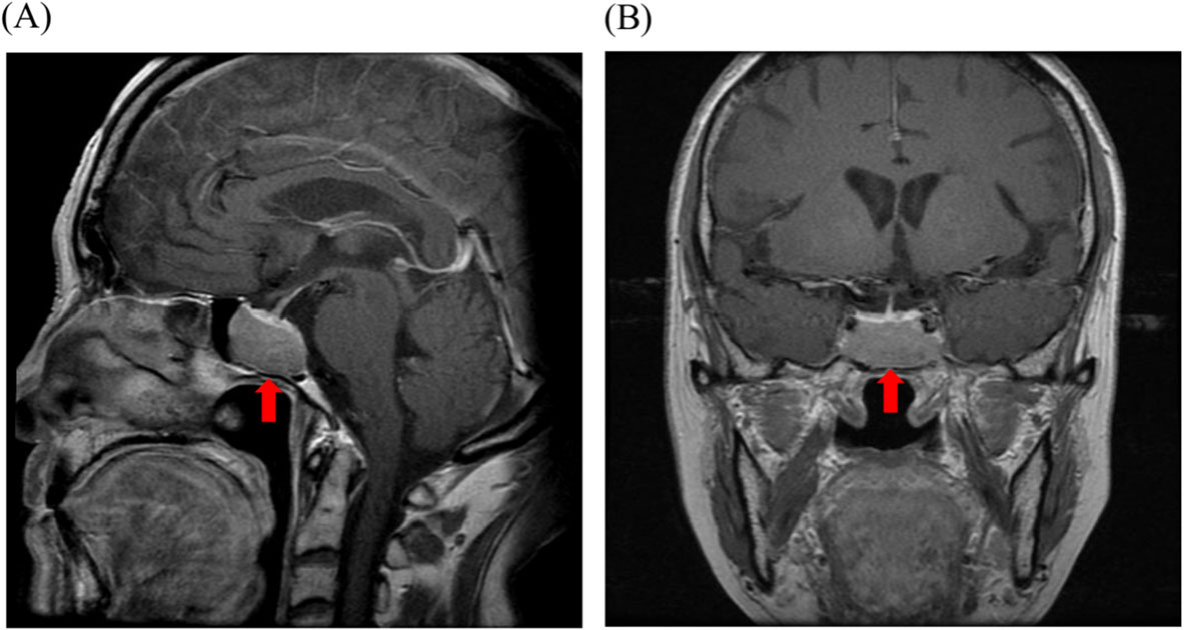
Intracranial magnetic resonance imaging (MRI). a Sagittal view of contrast-enhanced T1-weighted MRI. b Coronal view of contrast-enhanced T1-weighted MRI. red arrows: pituitary adenoma
